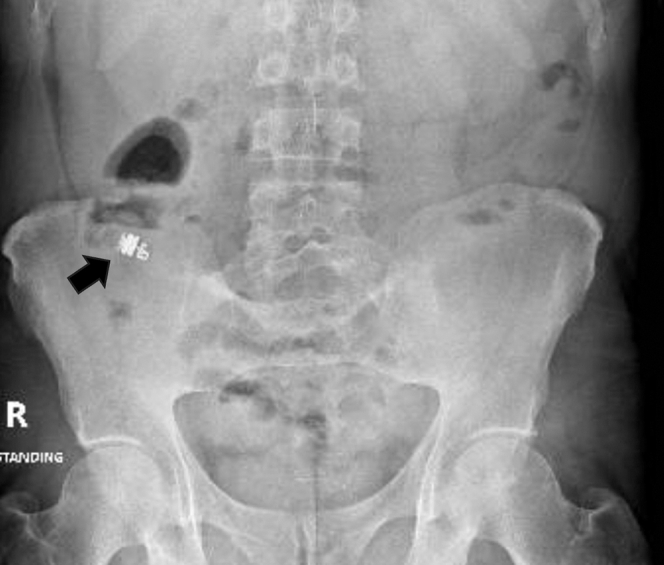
Abdominal radiograph performed after endoscopic stricture therapy showed the capsule retained in the small bowel located in the low-right quadrant (*black arrow*).
